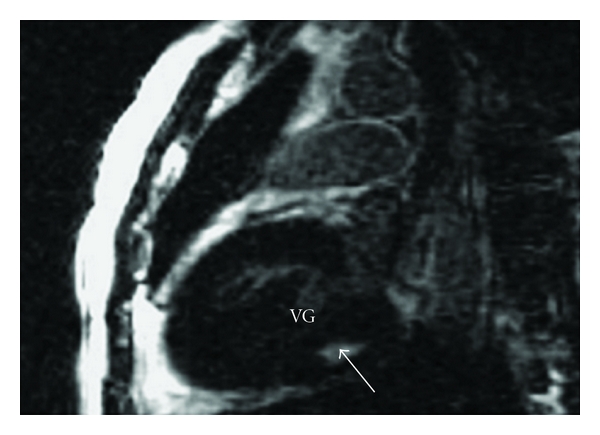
Cardiovascular magnetic resonance T1-weighted sequence obtained two weeks after discharge showed hyperintensity of the posterior lateral wall of the basal left ventricle. LVEF was 57%.
